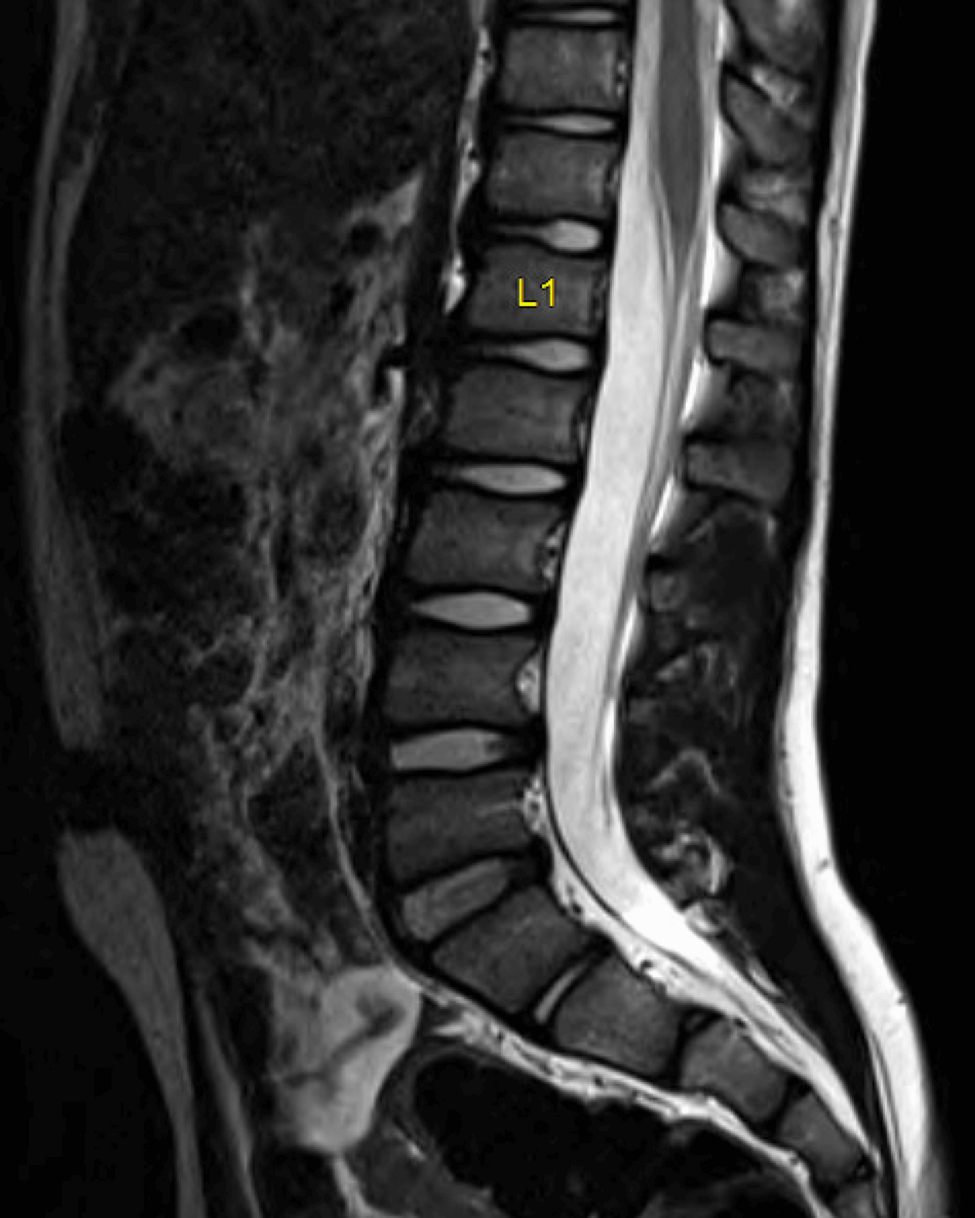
Lumbar MRI findings. A sagittal lumbar T2 MRI without abnormalities. The conus medullaris terminates at the level of the mid-body of L1, allowing adequate localization of the single-level laminectomy required to access the cauda equina allowing for access to the lumbar nerve roots.
